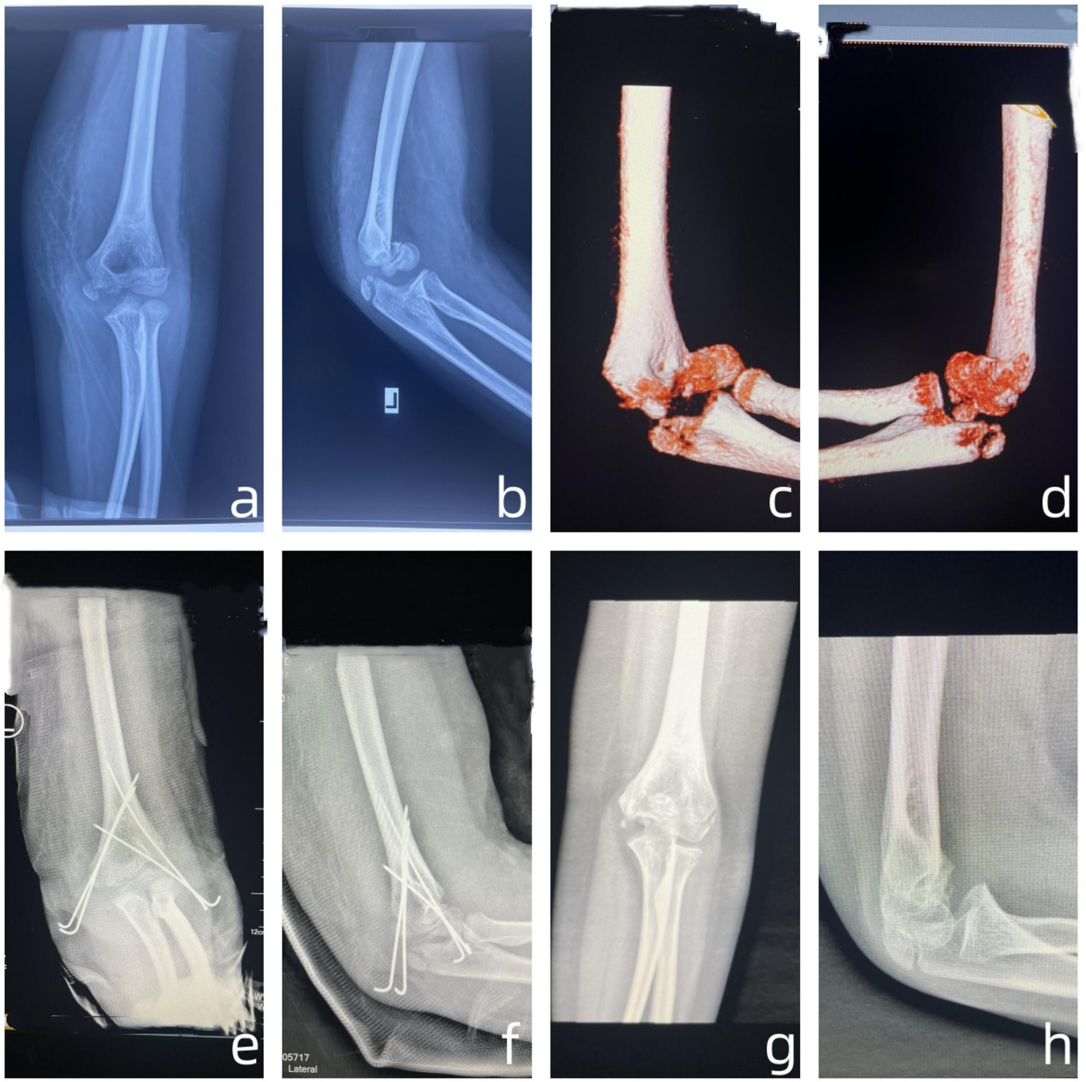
(a) One 10 years boy, sports injuries, 1 day after injury the Anteroposterior radiographs; (b) 1 day after injury the Lateral radiographs; (c) 1 day after injury the Anteroposterior Three dimensional CT; (d) 1 day after injury the Lateral Three dimensional CT; (e) 1 day post operation the Anteroposterior radiographs; (f) 1 day post operation the Lateral radiographs; (g) two months post operation the Anteroposterior radiographs; (h) two months post operation the Lateral radiographs.
